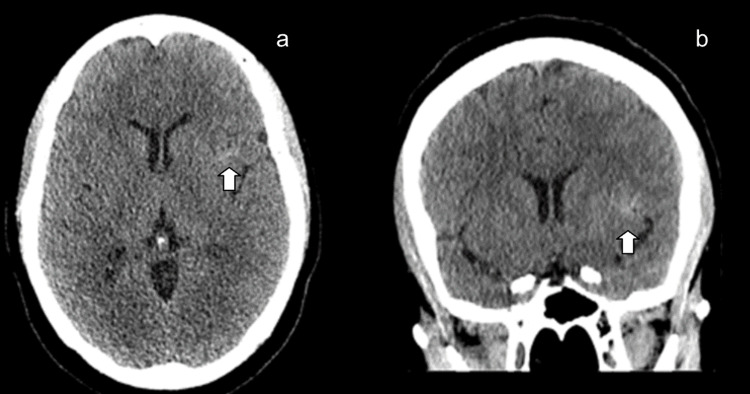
Computed Tomography (CT) of the Head Without Contrast Impression: Acute left subarachnoid hemorrhage in the left frontotemporal region without significant midline shift or mass effect (white arrow). (a) Axial view (b) Coronal view.
